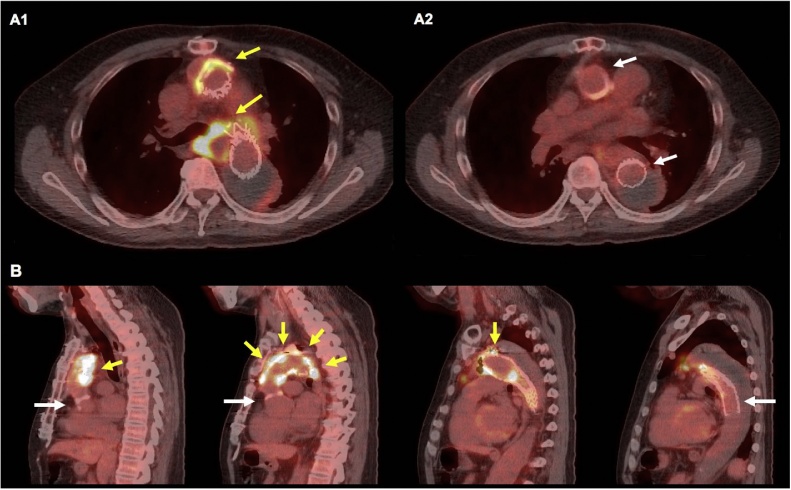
Preoperative ^18^F-fluorodeoxyglucose positron emission tomography/computed tomography (^18^F-FDG-PET/CT) findings (A; axial, B; sagittal view). Significant FDG uptake at the lesions with yellow arrows, while abnormal uptake was not observed at the sites with white arrows.
